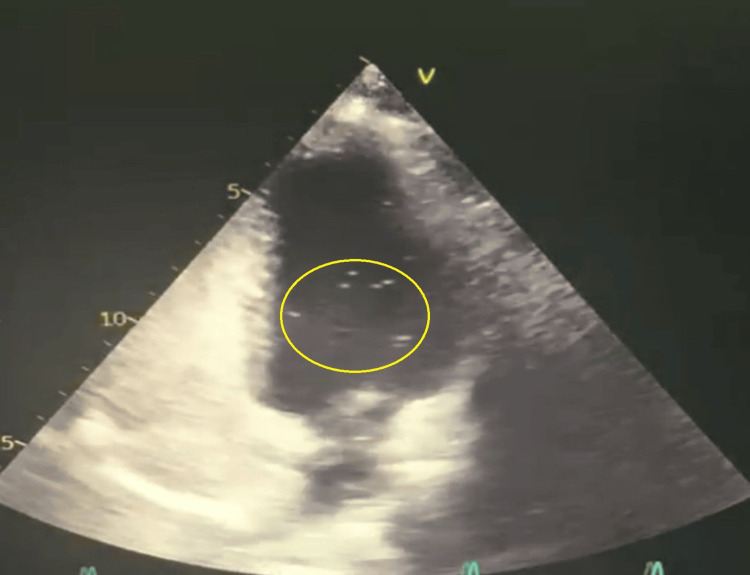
Bubble-contrast transthoracic echocardiogram (TTE) demonstrating an intracardiac shunt. Microbubbles are visible in the left atrium shortly after right atrial opacification, consistent with right-to-left shunting. The positive findings are highlighted with a yellow circle.
